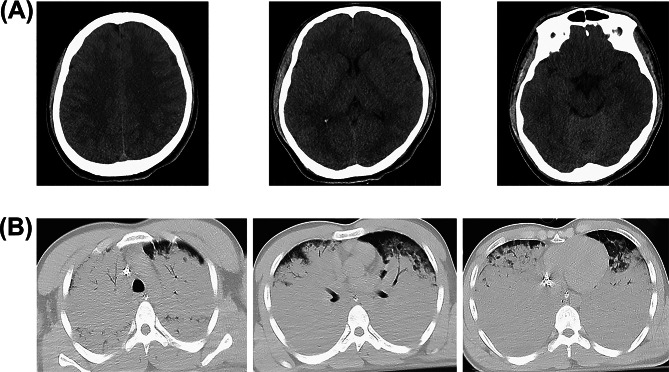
Computed tomography of (a) head and (b) chest on admission. a. Corticomedullary junction of the brain is clearly defined. b. Pulmonary edema or atelectasis in almost all areas of the lungs
